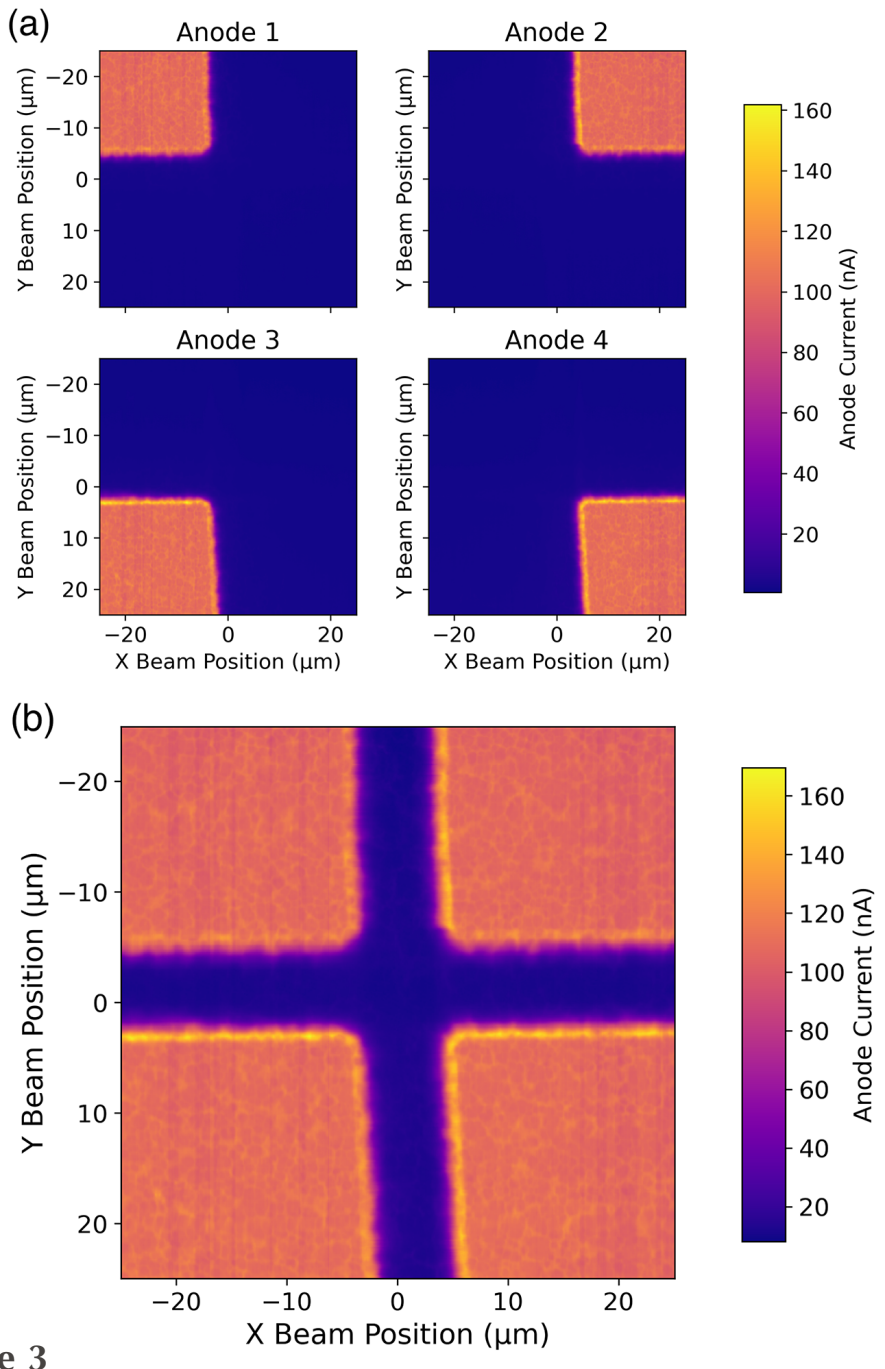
(*a*) Current measured on the individual sensor electrodes (Anode 1, Anode 2, Anode 3, Anode 4) when scanning an 8 keV X-ray beam on its surface. (*b*) Total current measured on the sensor computed as the sum of the individual electrode currents.
